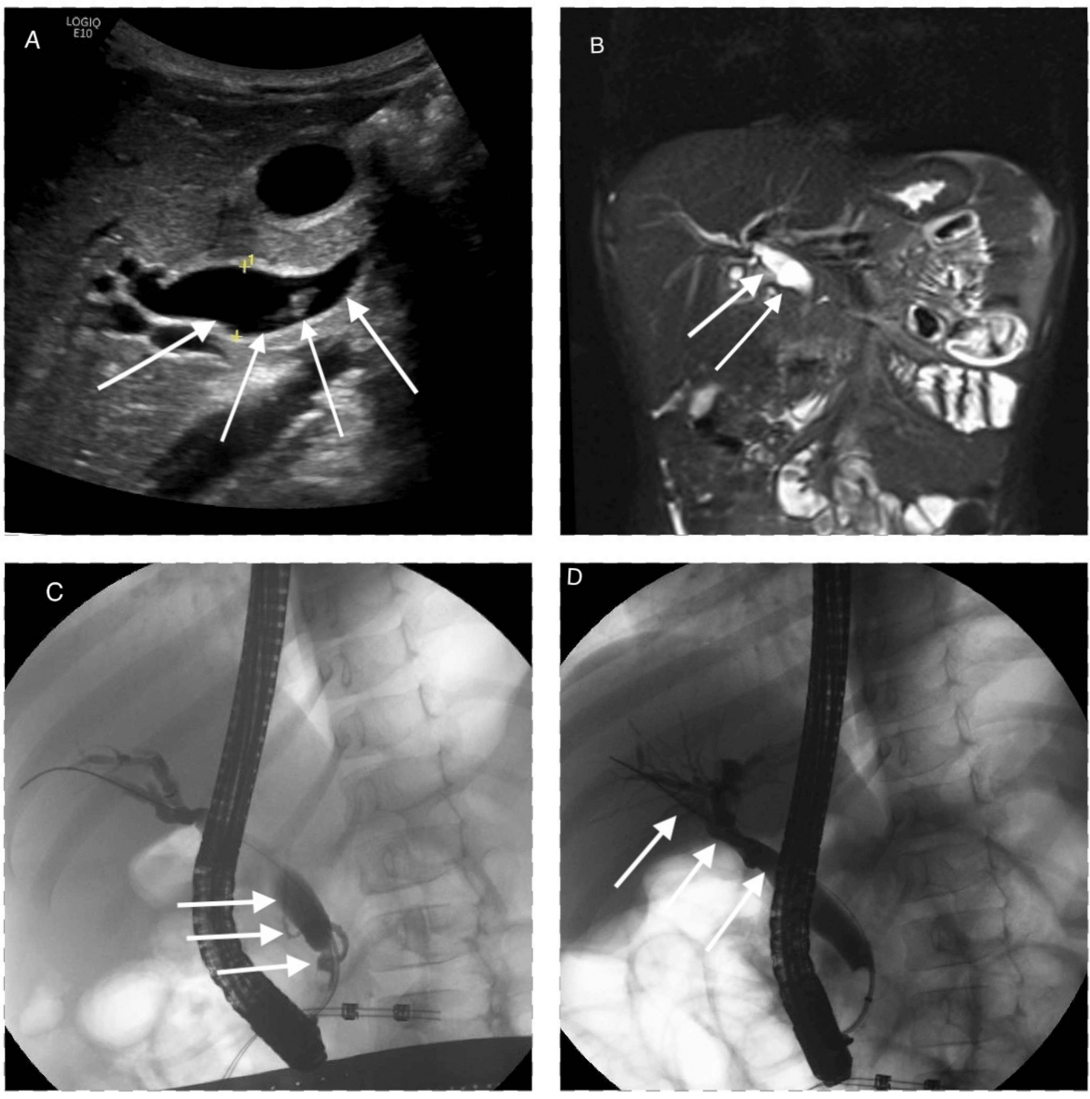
Radiographic imaging of dilated common bile duct, APBJ, and choledochal cyst highlighted by arrows. (A) Ultrasound with evidence of fusiform common bile duct dilation with choledocholithiasis; (B) coronal view of magnetic resonance imaging (MRI) of the abdomen with common bile duct dilation; (C) fluoroscopic imaging during ERCP with evidence of an aberrant pancreatic biliary junction; (D) fluoroscopic imaging during ERCP with identification of Type IA choledochal cyst. APBJ: anomalous pancreaticobiliary junction
